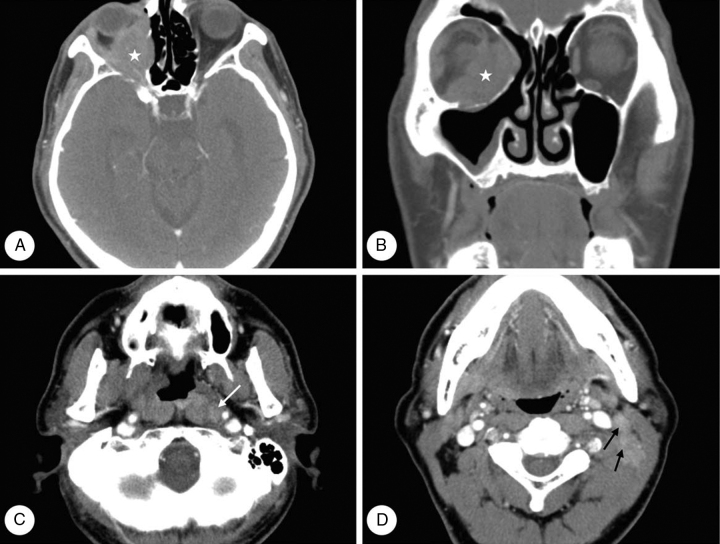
Computed tomography (CT) images of case 2. Axial (A, C, D) and coronal (B) images reveal a soft tissue mass () in the extraconal and intraconal space of the right orbit. Moreover, asymmetry of the left nasopharynx and metastatic lymph nodes is noted in the level II (black arrow) and retropharyngeal areas (white arrow) of the left neck.
